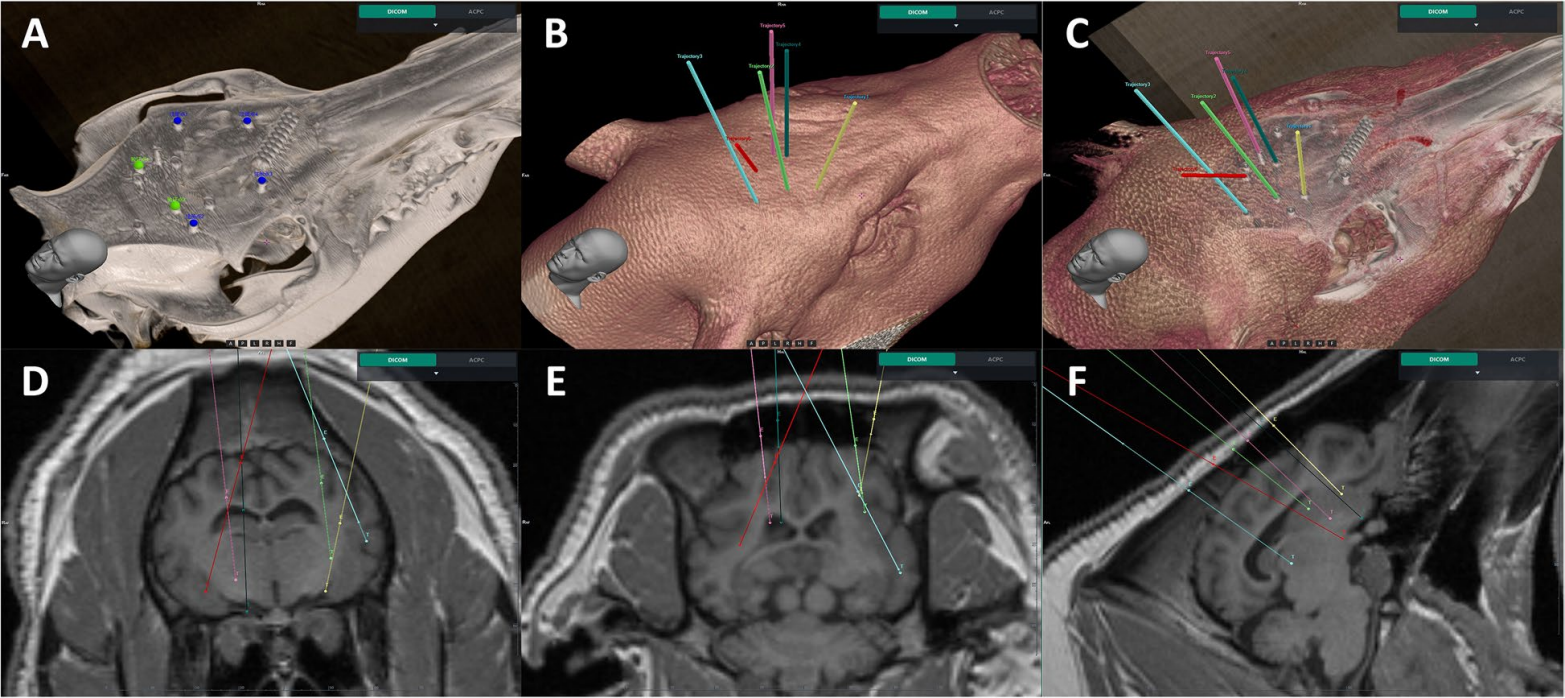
Markers were automatically segmented from preoperative CT image and confirmed by surgeon. A Four markers (blue) for registration and two markers (green) for verification. B Entry and target points for the electrodes. C 3D model reconstructed based on fusion images to check trajectories. D, E, F Transverse section, coronal section, and sagittal section of the electrode placement
